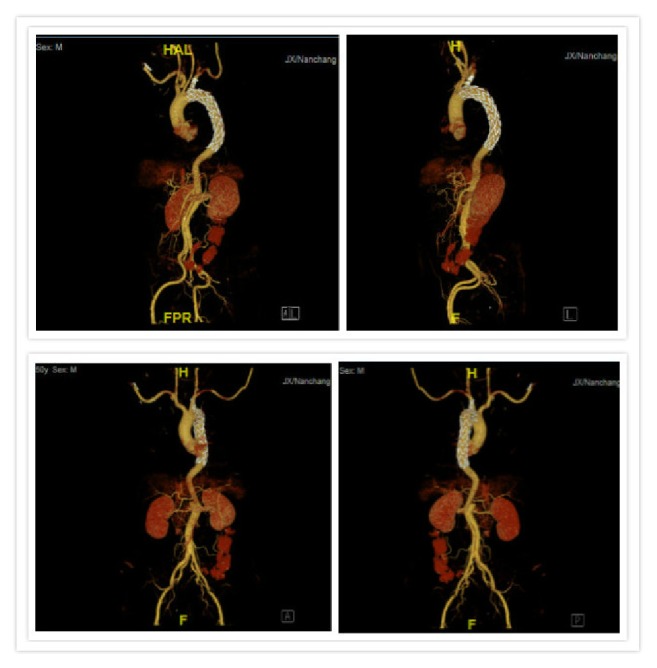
Follow-up CTA on 12 months after operation. Complete thrombus formation of the pseudoaneurysm and patency of the stents.
